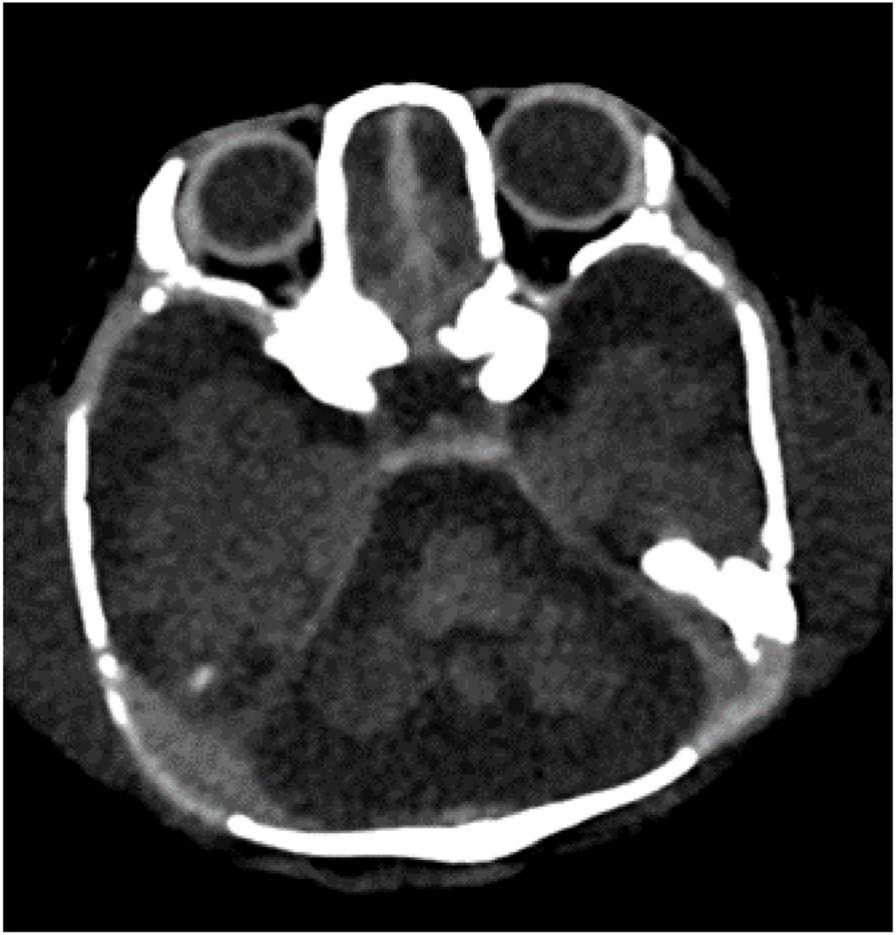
Postmortem head CT imaging of the patient. The CT imaging showed hypoplasia/atrophy of the cerebellum and enlarged cisterna magna
